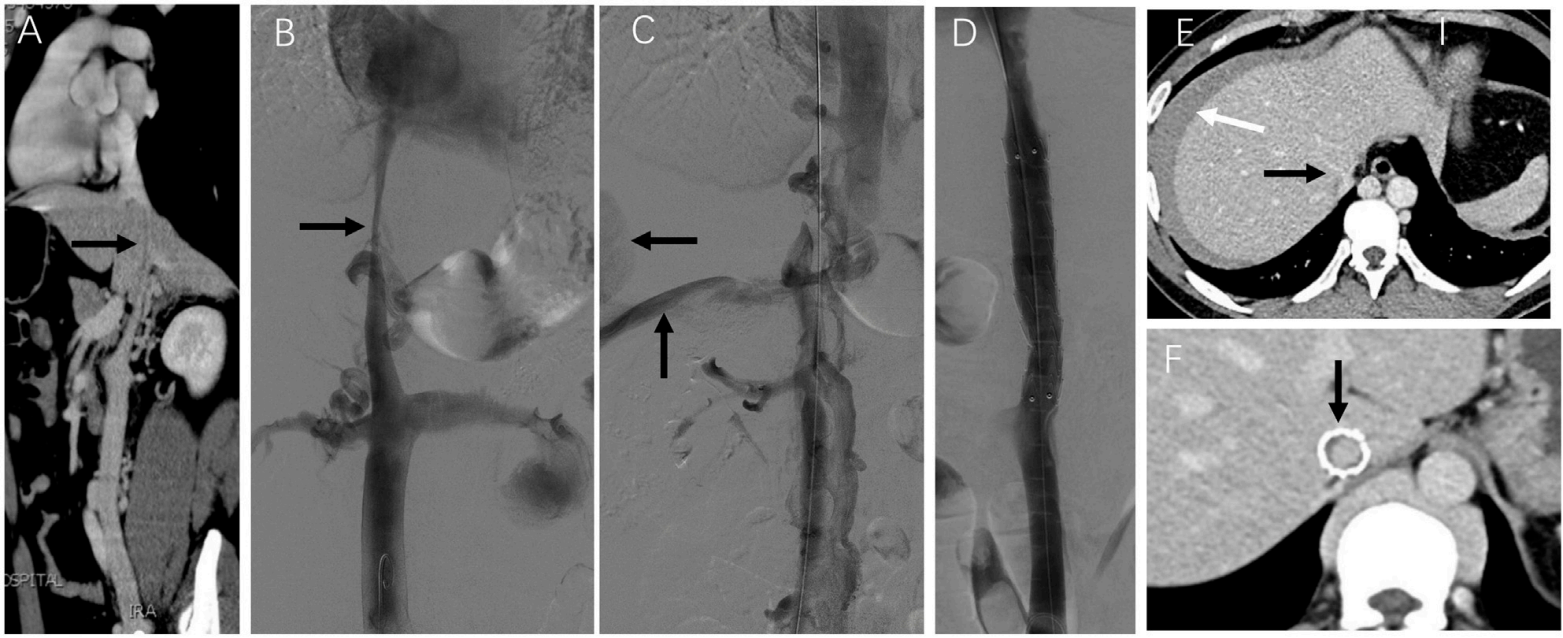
Emergency treatment and long-term observation of IVC stenosis. Note: After 20 months, the patient in case 3 experienced bloating once again. (A) Subsequent CT angiography performed 2 years later revealed the presence of severe stenosis in the IVC (indicated by arrows). (B) Despite dilatation using a 20 mm balloon, angiography still demonstrated considerable stenosis in the IVC (indicated by arrows). (C) During the dilation procedure using a more enormous 26 mm balloon, the patient reported experiencing intense abdominal and back pain. Following the angioplasty with the 26 mm balloon, there was a visible extravasation of contrast agent (indicated by arrows) and occlusion of the IVC due to abundant collateral circulation. The patient rapidly experienced a drop in blood pressure, which indicated a rupture in the IVC. (D) To address the rupture, a covered stent measuring 16*60 mm was successfully placed within the IVC between the hepatic and renal veins. This placement restored the patency of the IVC without any contrast agent leakage. The patient’s condition improved following transfusion and fluid replacement therapy, resulting in discharge from the hospital on the 7th day after the surgery. (E) A CT scan 7 days later showed unobstructed hepatic veins and IVC (indicated by black arrows), with an intra-abdominal hematoma (indicated by the white arrow). (F) In the first year following stent placement, a CT scan confirmed the patency of the stented area in the IVC with no signs of stenosis (indicated by arrows).
